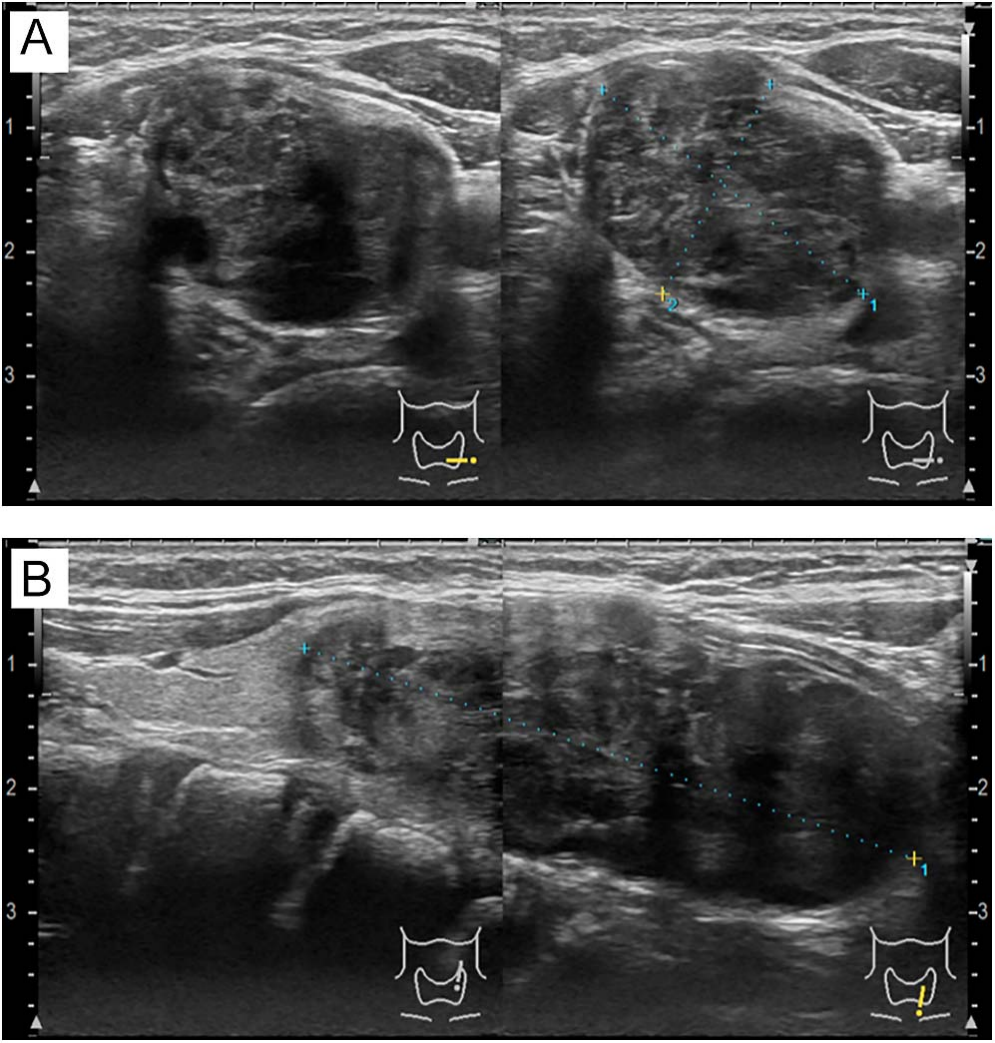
Cervical ultrasonography showed a 50 mm large heterogeneous mass extending from the left lobe of the thyroid gland to the mediastinum. (A) Transverse view. (B) Longitudinal view.
